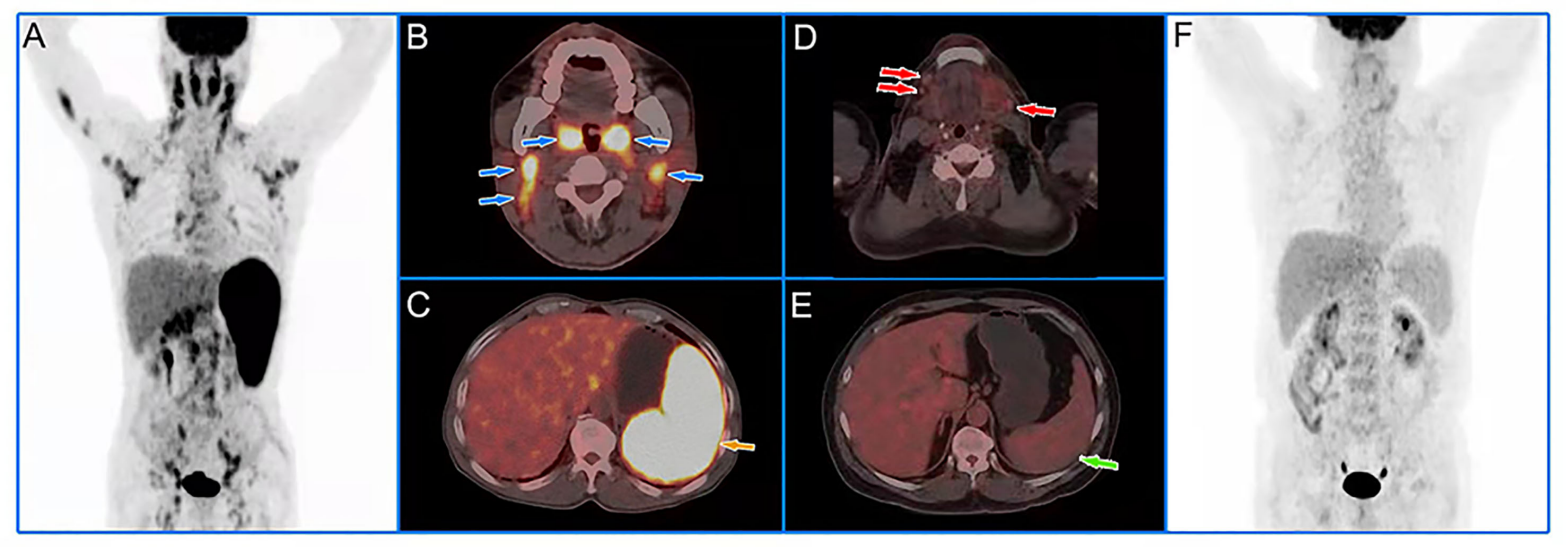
Representative cases of baseline ^18^F-FDG PET/CT images. A 60-year-old male patient with low HI had extensive lymph node lymphoma invasion and lymphoma infiltration into the oropharynx and spleen (A). The blue and yellow arrows represented the oropharynx, cervical lymph nodes (B), and spleen (C), respectively. The lesions had an SUV_max_ of 11.09, an SUV_mean_ of 7.10, and a HI of 1.56. We followed up for 39 months and the patient had no recurrence. A 55-year-old male patient with high HI had extensive critical-sized lymph node lymphoma invasion and no lymphoma infiltration into the organs (F). The red and green arrows represented the submaxillary lymph nodes (D) and spleen (E), respectively. The lesions had an SUV_max_ of 3.35, an SUV_mean_ of 1.13, and a HI of 2.96. The patient developed local recurrence after 29 months of follow-up.
